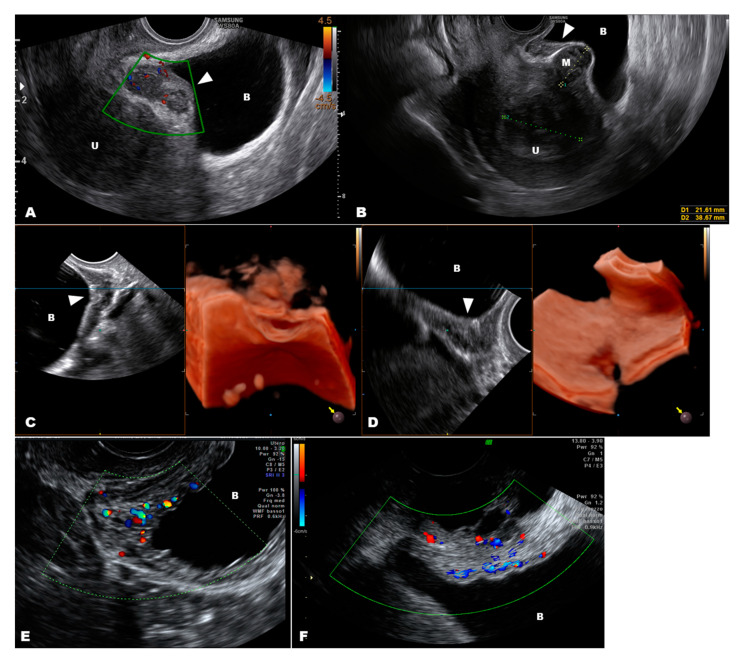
A typical endometriosis nodule (arrow) of the bladder (A); figure (B) shows a flat nodule of endometriosis (arrow) with a subserosal myoma that distorts the bladder dome; a flat endometriosis nodule (arrow) of the bladder that can be seen at 3D ultrasound only when cut (C) while the internal surface of the bladder appears regular (D); figure (E) shows a thick bladder wall due to cystocele and figure (F) in case of recurrent cystitis. Abbreviations: U, uterus; B, bladder; M, myoma.
